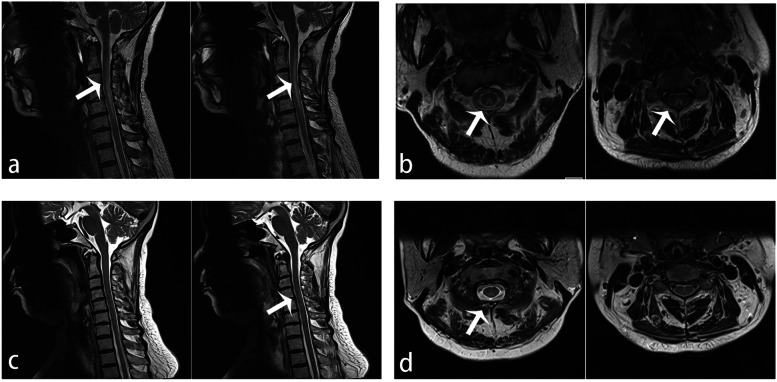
Spinal cord MRI. a Sagittal T2-weighted imaging showed long segmental hyperintensities from C2 to C6 of the spinal cord. b Inverted “V-sign” hyperintensity on axial T2-weighted imaging within the dorsal cervical spinal cord. c After 3 months of continuous immune therapy, there was a decreased signal on axial T2-weighted imaging, both horizontally and longitudinally. d After 3 months of continuous immune therapy, the inverted “V-sign” hyperintensity faded on axial T2-weighted imaging
